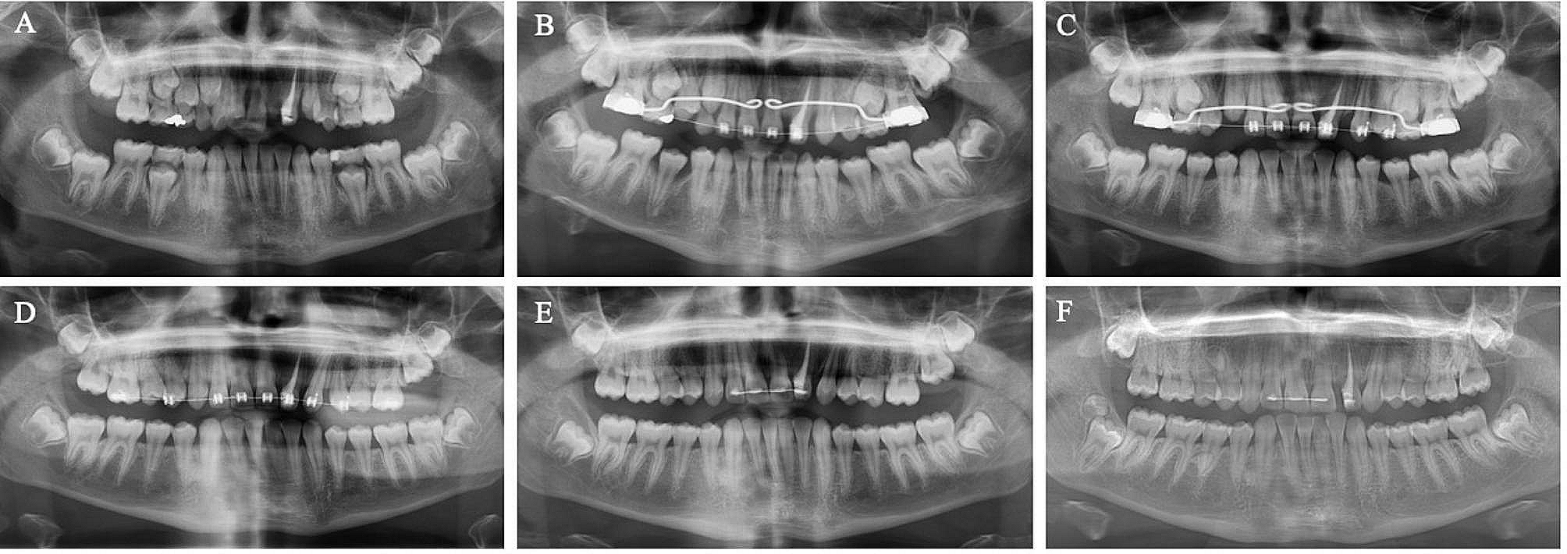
Follow-up panoramic radiographs of Case 2 with a concrescent left lateral incisor. (A–F) panoramic radiographs of 6, 10, 12, 18, 24 months, and 6 years after hemisection, respectively
